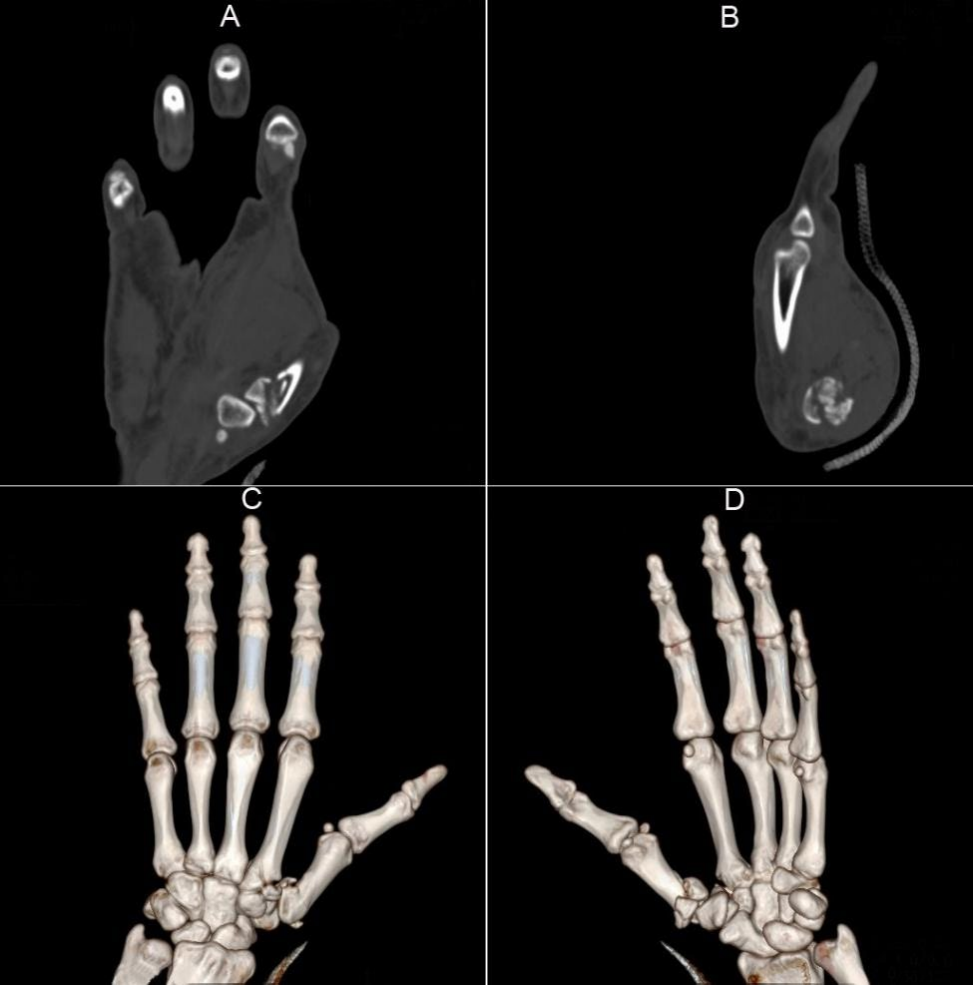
Representative computed tomography images of patients with communited first metacarpal bone base fracture. (A and B) The fracture of the first metacarpal bone base led to comminuted articular surface, partial articular surface collapse, and dorsal dislocation of the first carpometacarpal joint. (C and D) The fracture of the first metacarpal bone base led to that the joint was displaced to the palmar side and was crushed into three pieces
